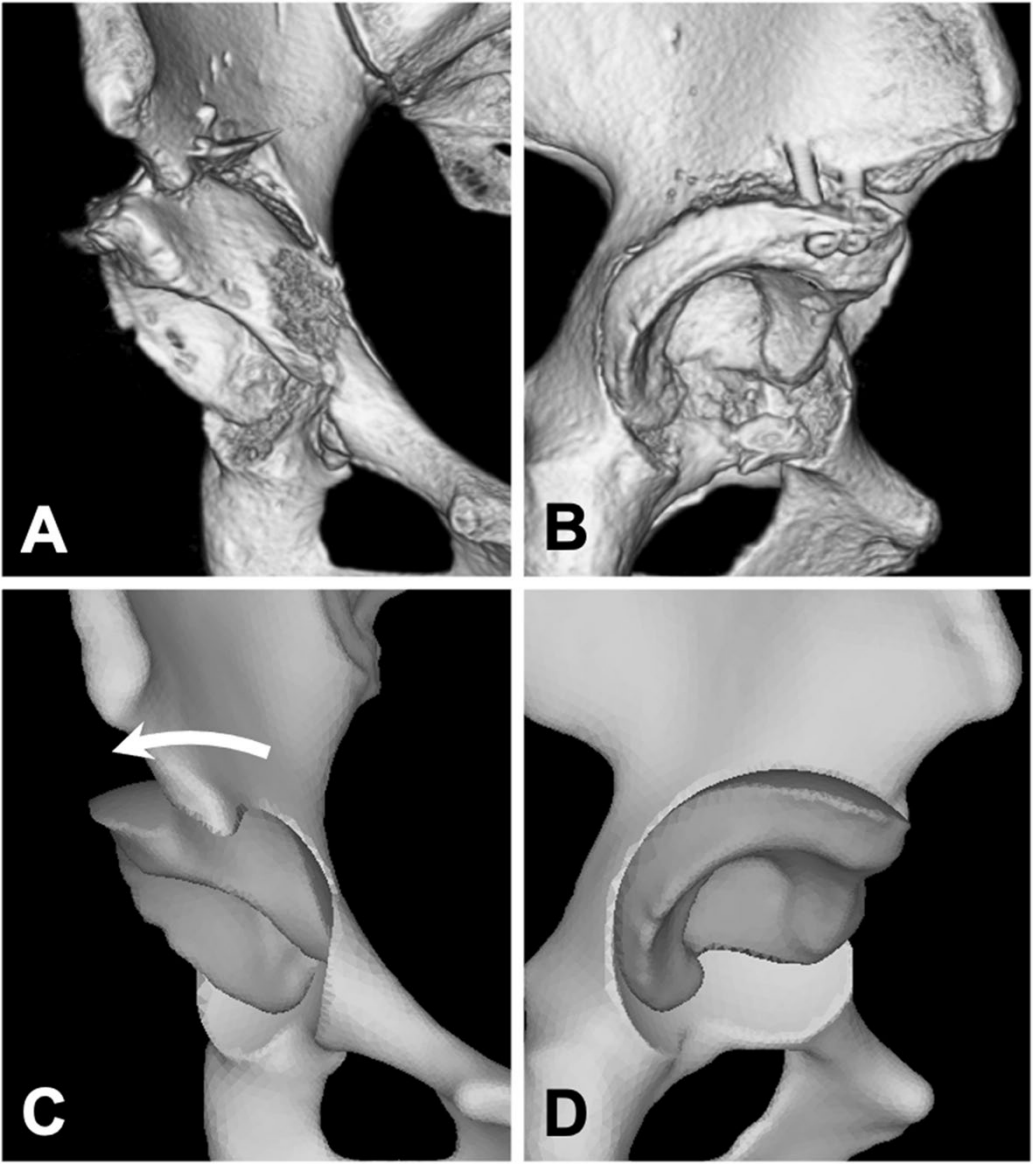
A Anteroposterior and B lateral views of a computed tomography image of a patient with a right dysplastic hip after transposition osteotomy of the acetabulum (TOA). C Anteroposterior and D lateral views of the 3D surface model created from CT images of the same patient after virtual periacetabular osteotomy mimicking TOA
